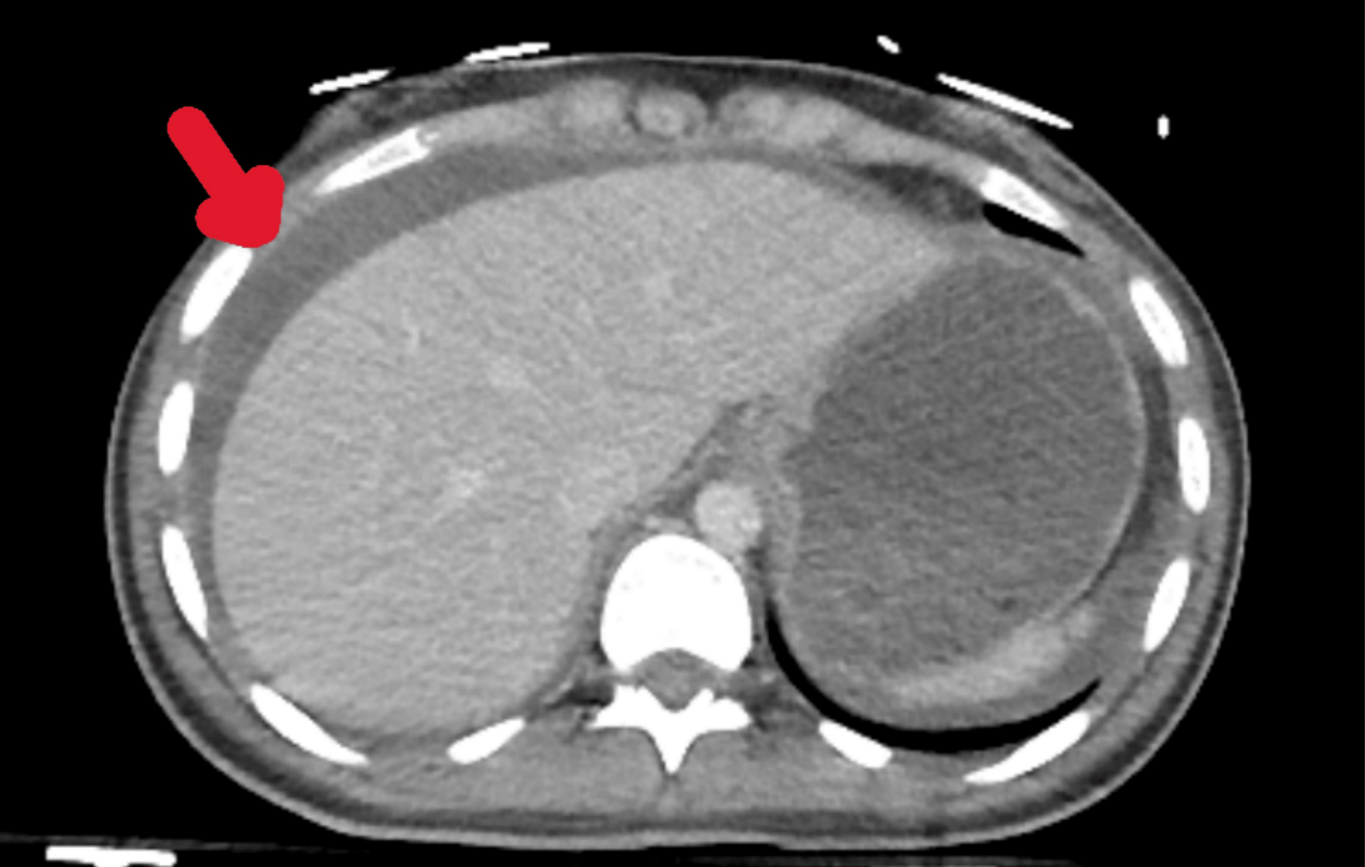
Computed tomography demonstrating diffuse hemoperitoneum (red arrow) without evidence of solid organ injury.
